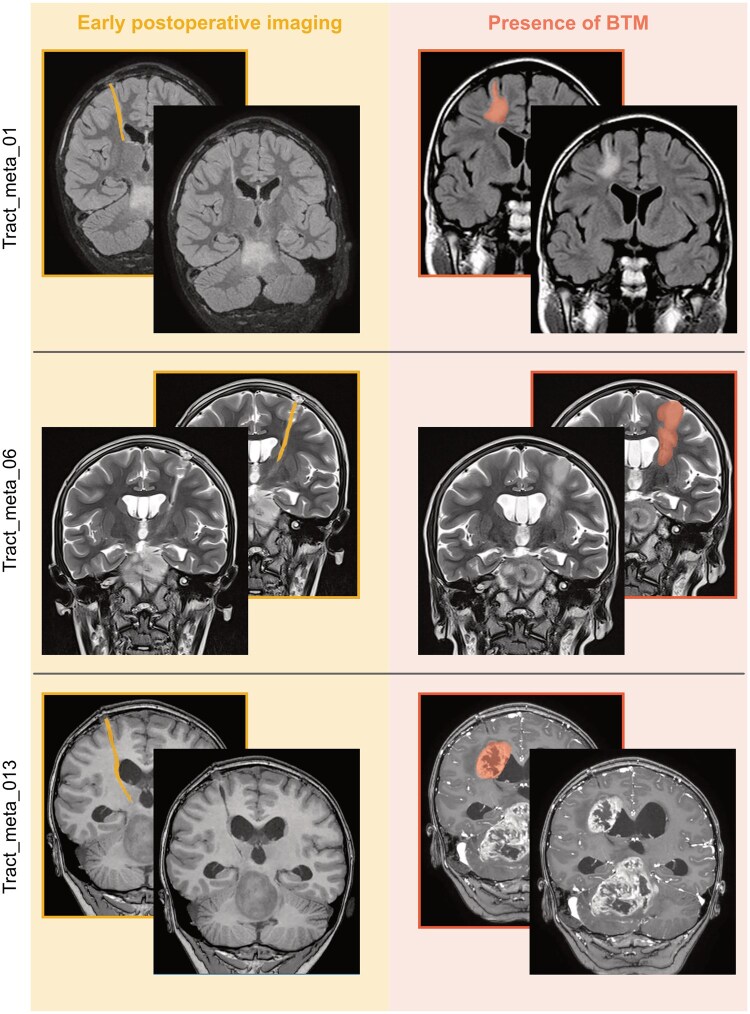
Representative examples of biopsy track metastasis (BTM). Postoperative magnetic resonance images are shown on the left (yellow) with the biopsy trajectory, and follow-up images after emergence of BTM are shown on the right (red). Color-overlay duplicates are provided to facilitate visualization of the biopsy trajectory and needle-track metastasis.
